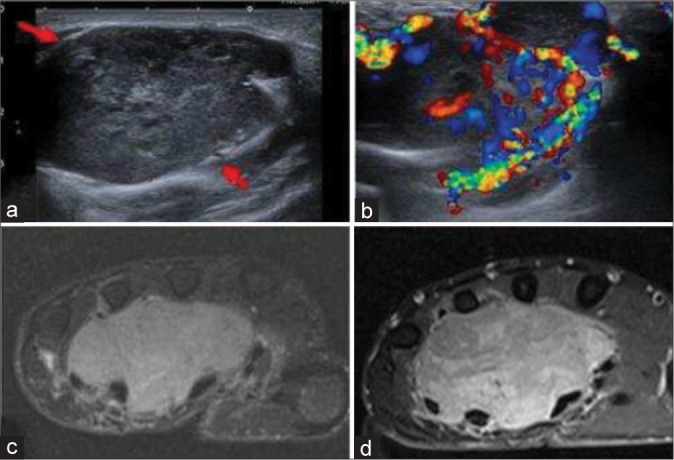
A 30-year-old woman with synovial sarcoma of hand who presented with slow-growing mass on hand. (a) Gray scale ultrasound image shows a well-defined solid and heterogeneous lobulated mass in volar surface of the right hand (red arrows). (b) Color Doppler shows significant color flow. (c) Fat saturated axial T2-weighted image showing hyperintense lobulated and insinuating mass, (d) with heterogeneous enhancement on post-contrast fat saturated T1-weighted axial image.
